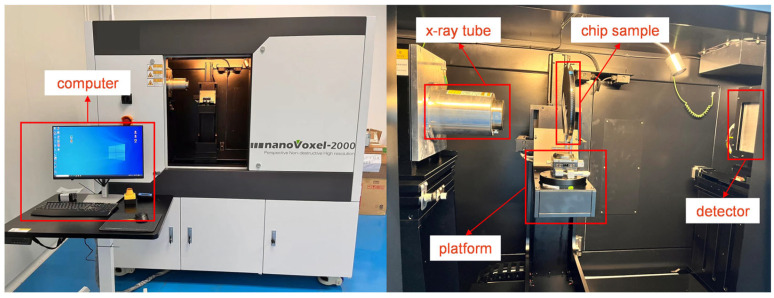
X-ray Image Data Acquisition Equipment.
